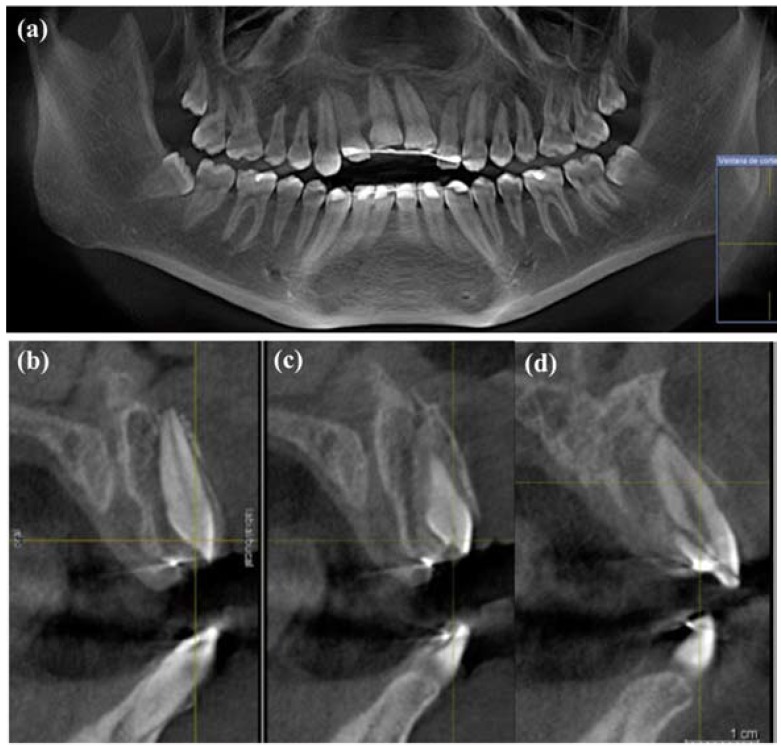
(a) The initial orthopantomography. (b) CBCT radiographic examination, showed an alveolar bone fracture and traumatic luxation in 1.1. (c) CBCT radiographic examination showed a fracture of the vestibular cortical bone. (d) CBCT radiographic examination showed a complicated crown-root fracture in 2.2.
